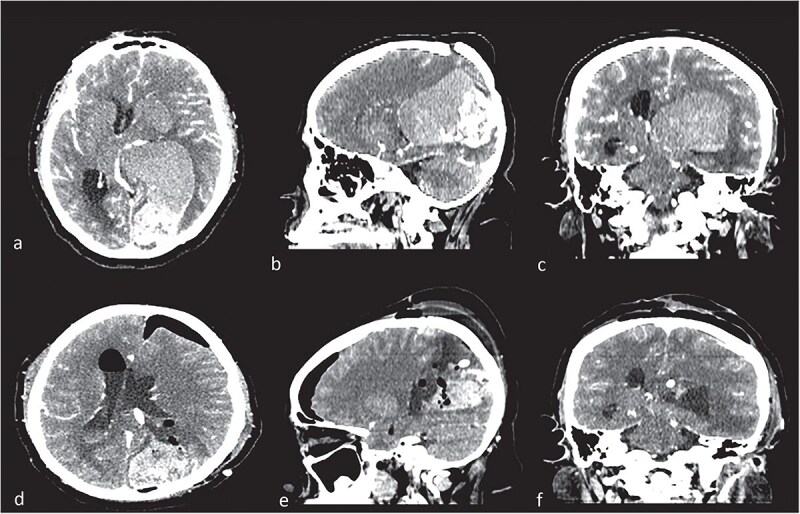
Contrast computed tomography (CT). (a–c) Intraventricular tumor, with a high volume, with the lateral ventricle shape. Different density in the tumor meaning intratumorally hemorrhage. (c–e) Control CT with resection of low-density lesion.
